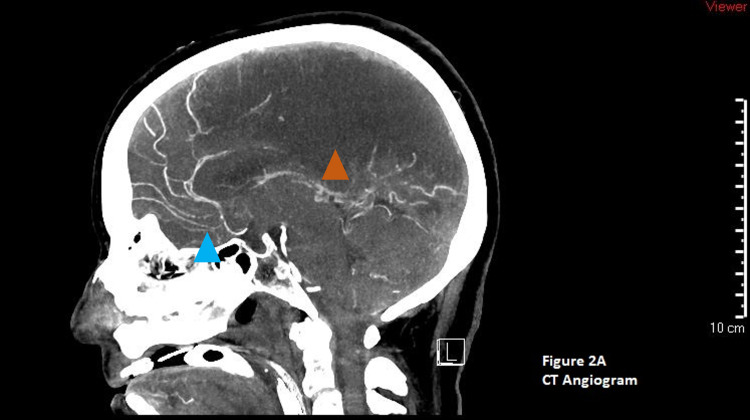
CT angiogram of the head. The blue triangle arrow shows normal blood vessels. The orange triangle highlights cerebral edema.
